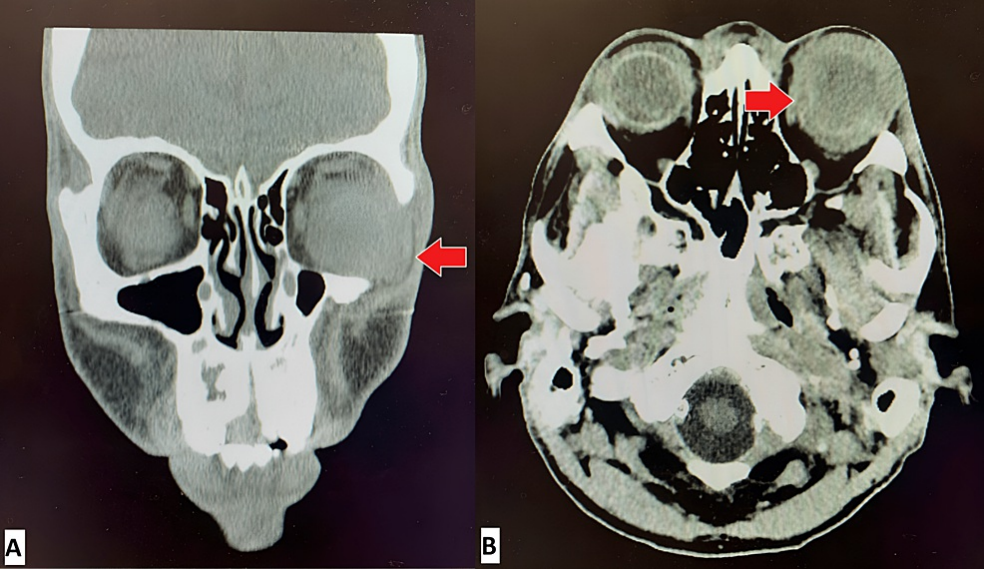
Patient’s head CT scan showing more left eye findings than the right Panel A: Head CT scan without contrast coronal view.  The arrow points to the left globe, showing an elongated and proptotic globe with scleral irregularity/discontinuity. The swelling extends into the post septal and intraconal space, with increased density within the posterior compartment and posterior aspect of the vitreous. Similar appearing, less severe findings are seen involving the right globe with circumferential swelling extending postseptally/intraconally with scleral irregularity and increased density within the posterior compartment. Panel B: Head CT scan without contrast axial view. The arrow points to the left globe, depicting left greater than right periorbital soft tissue swelling with fluid collections surrounding the globes more anteriorly. There is no evidence of thrombosis or superior ophthalmic veins or cavernous sinus thrombosis in this study.
